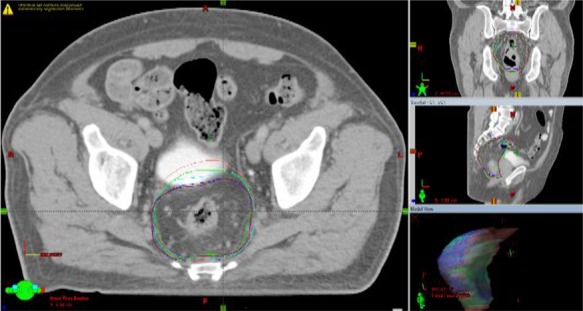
Changes in mesorectum contours on cone-beam CT images matched to planning CT scans (P-CT, CB-CT 1, CB-CT 2, CB-CT 3, and CB-CT 4)
